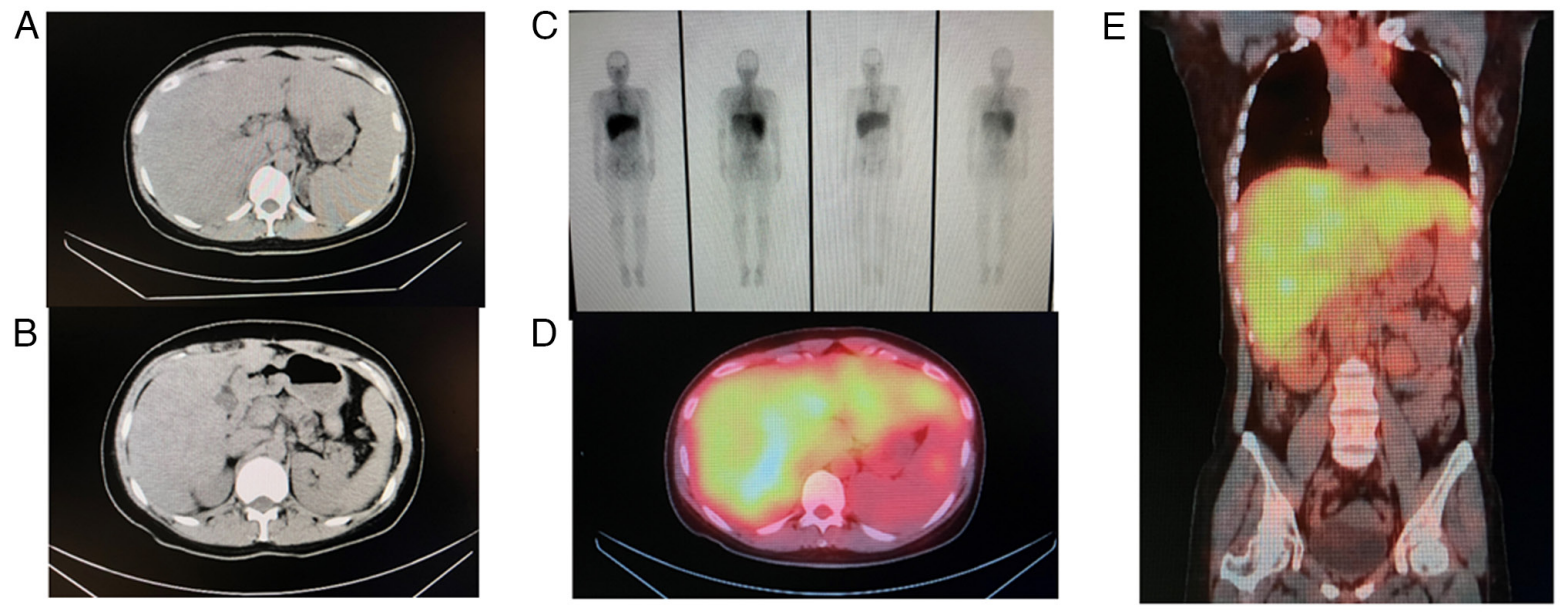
Pre-treatment CT scan and gallium-67 scintigraphy. (A and B) An abdominal CT scan revealed extensive hepatosplenomegaly. (C-E) Gallium-67 scintigraphy demonstrated a marked uptake in the liver and uptake in the mediastinal, inguinal and external iliac lymph nodes. CT, computed tomography.
